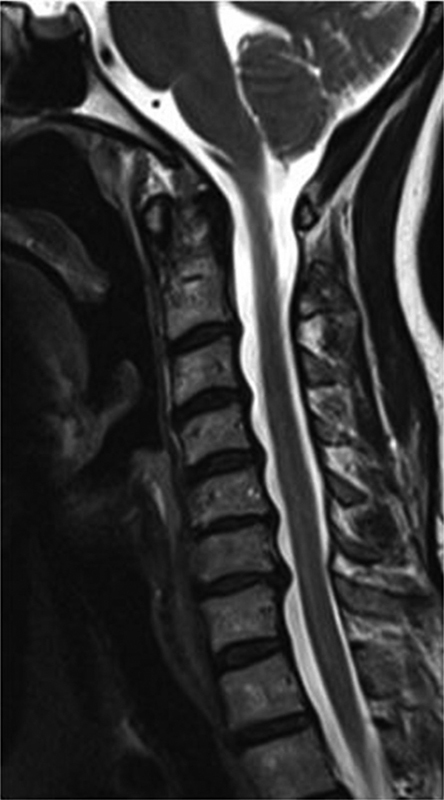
Cervical spine sag T2-weighted image postantituberculous therapy showing resolution of cord edema.
